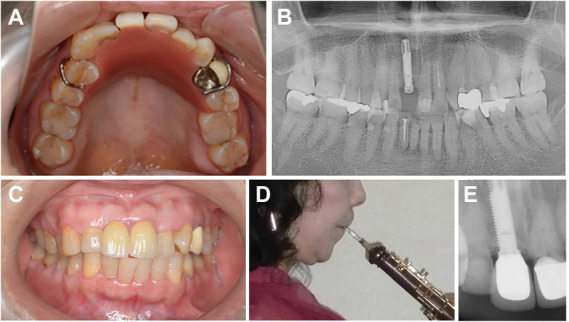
(A) Occlusal view with the removable prosthesis placed (19.7.2012). (B) Panoramic radiograph with the dental implant inserted (19.7.2012). (C) Intraoral view with the final prosthesis in place (7.3.2013). (D) Lateral view of the patient with the oboe in place (7.3.2013). (E) Dental radiograph after 8 years observation (2.9.2021).
